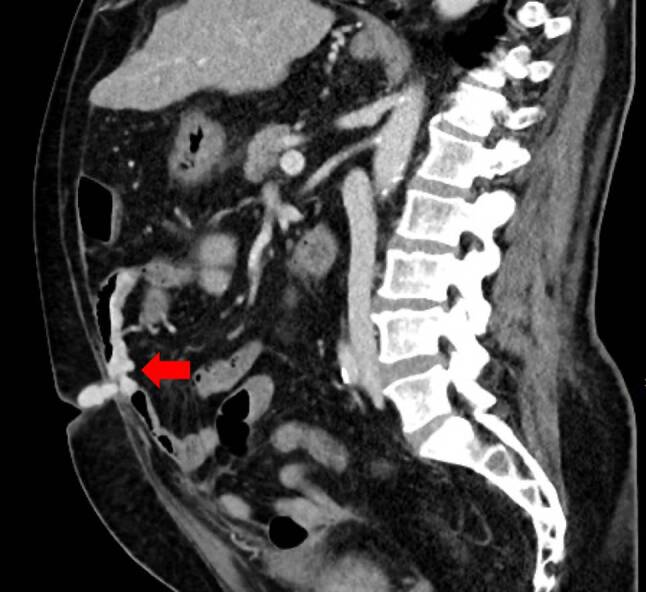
Venous “pooling” during contrast-enhances computed tomography as a sign of luminal bleeding into the jejunum in sagittal plane (*arrow*)
